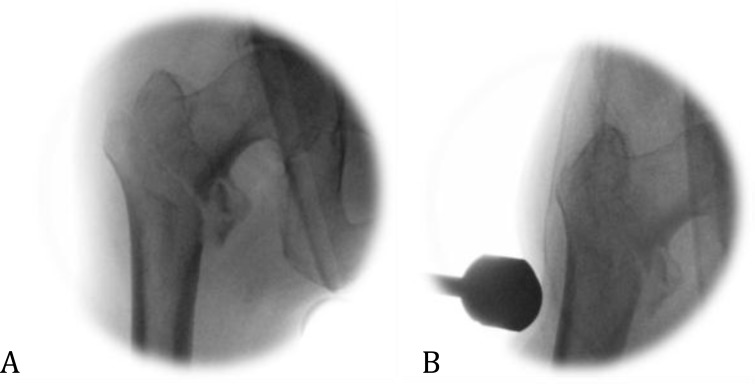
AP radiographs of a right hip showing (A) a laterally displaced fracture (B) the fracture reduced with lateral pressure applied firmly over the fracture site.
